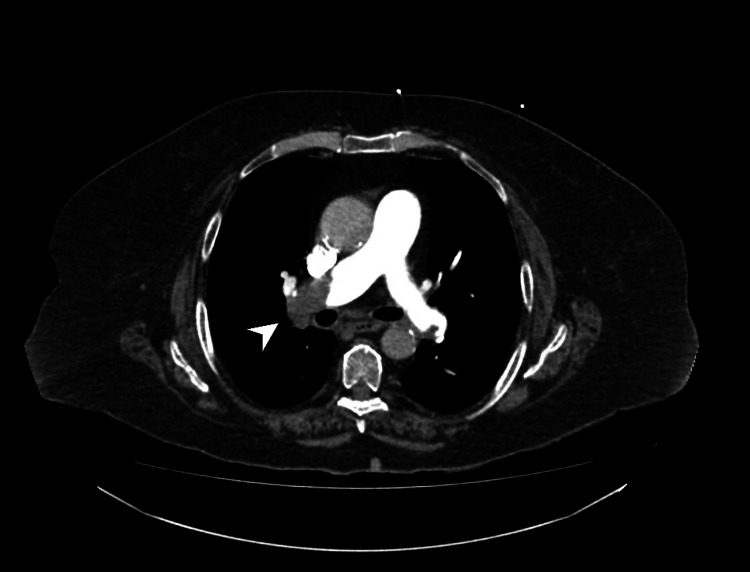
Chest CT confirming acute pulmonary embolism (white arrowhead) CT: computed tomography
